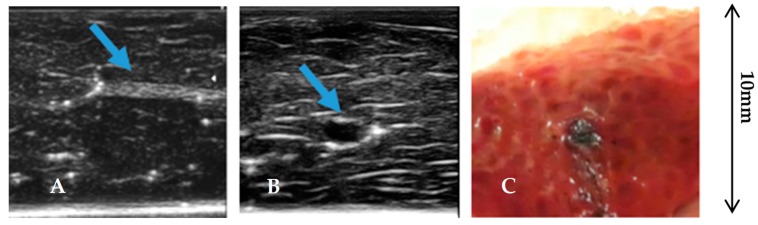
B-scan image of the internal structure of the liver sample (A) using a linear array ultrasound transducer SL3116. (B) B-scan image of the liver acquired over a 2 mm agar inclusion using the same linear transducer; (C) picture of the liver slice obtained dividing the tissue into two pieces in correspondence of the inclusion.
